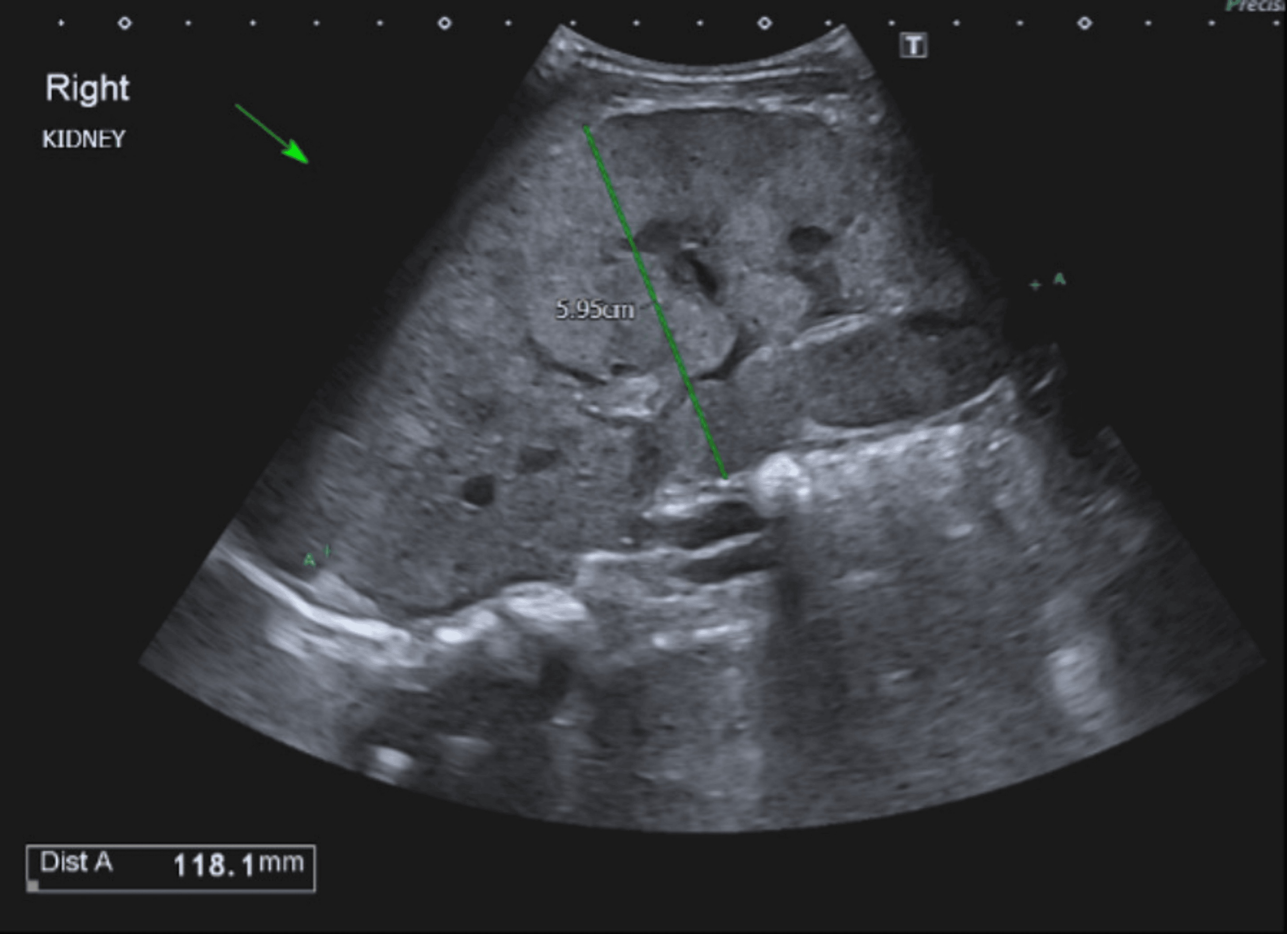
Abdominal ultrasonography of right kidney with largest width measurement of 5.95 cm and length (Dist A) of 11.81 cm
